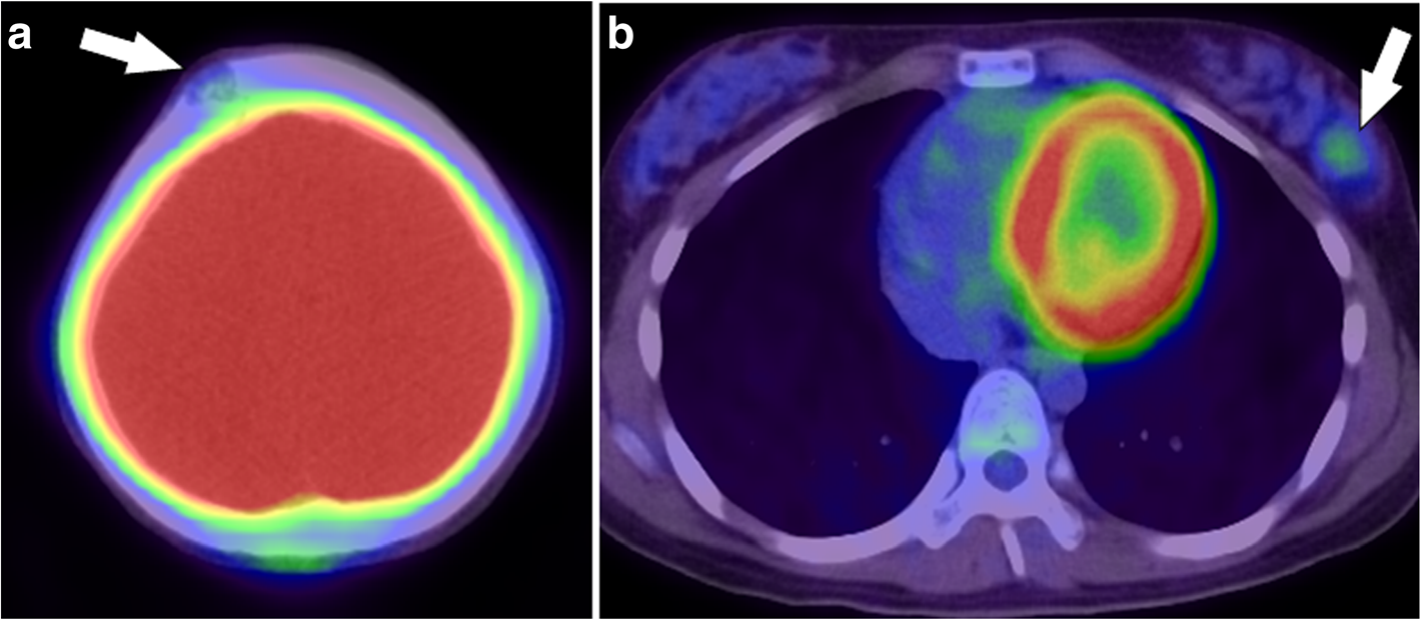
FDG-PET / CT image findings: Accumulation of fluorodeoxyglucose (FDG) was observed in the right front of the skull (standardized uptake value (SUV)-max 2.8) (a) and left breast (SUV-max 2.4) (b) using FDG-positron emission tomography (PET) / computed tomography (CT)
